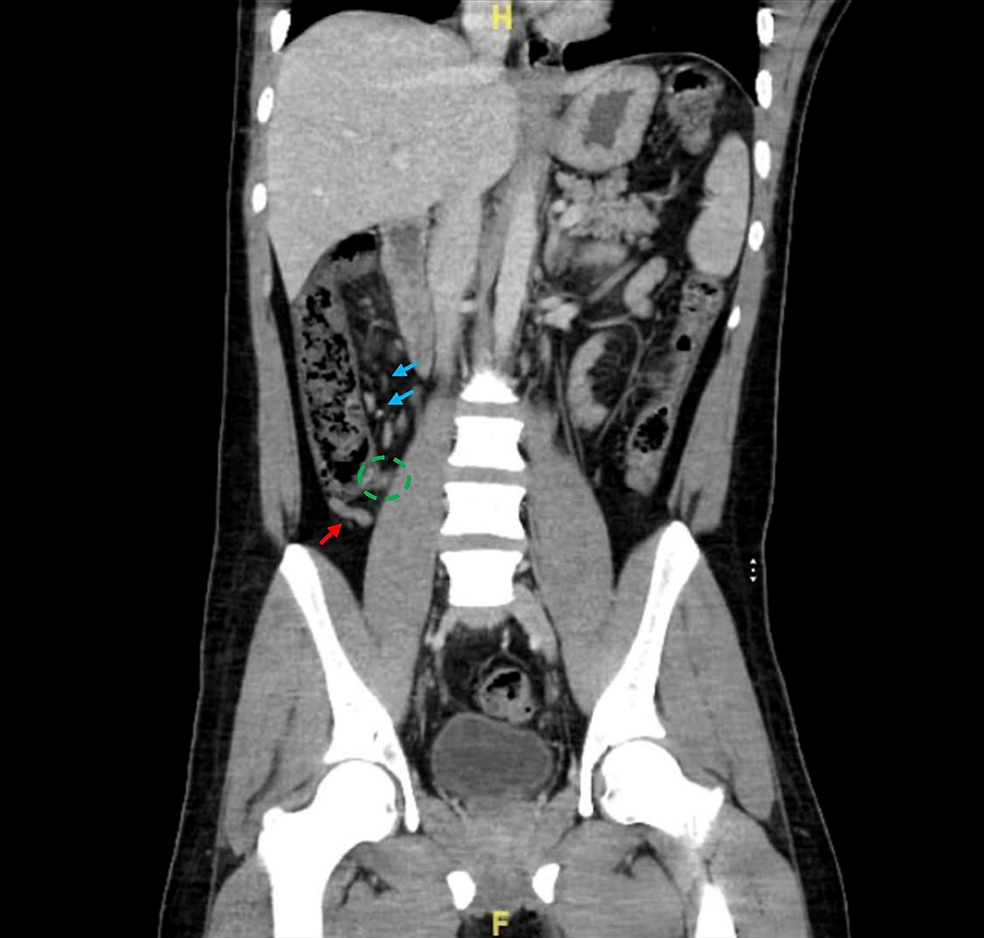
CT of the abdomen with contrast The appendix (red arrow) is seen at the right iliac fossa with a normal caliber of about 7mm, minimal surrounding fat stranding (green dashed circle), and small mesenteric lymph nodes (blue arrows), consistent with an early acute appendicitis diagnosis.
